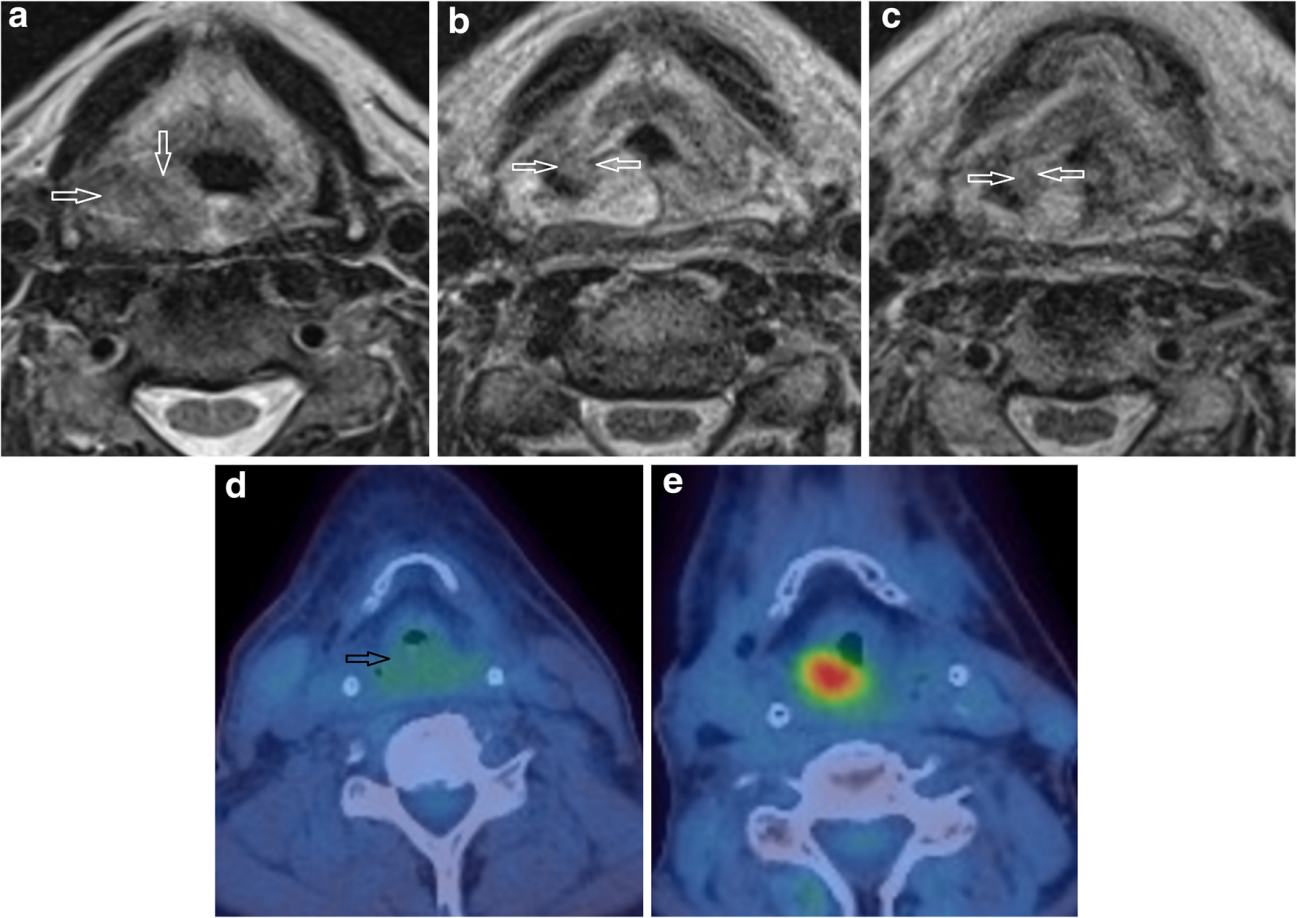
Right piriform carcinoma which recurred at 2-year follow-up. a–c T2w axial images on (a) pre-treatment, (b) 6-week post-treatment and (c) 12-week post-treatment MRI. The T2 morphology scores (lesions indicated by open white arrows) were 2 on pre-treatment, 1 on 6-week post-treatment and 1 on 12-week post-treatment MRIs. d 18-FDG PET-CT at 12 weeks post-treatment did not reveal any focal uptake but e subsequent 18-FDG PET -CT demonstrates focal uptake with a time to recurrence of 215 days
